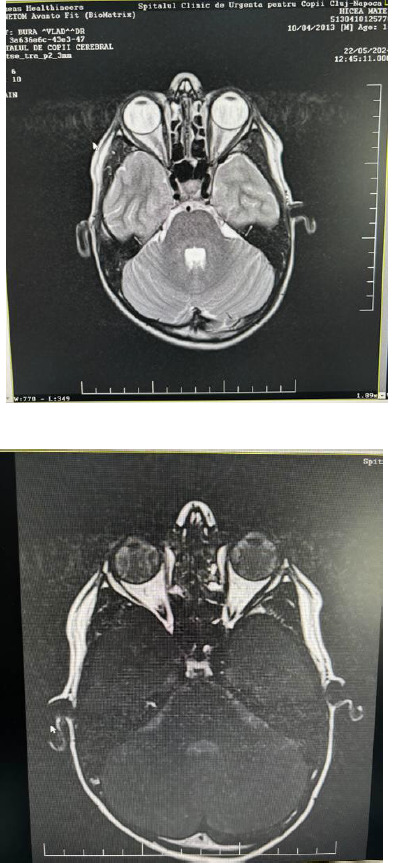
The nuclear magnetic resonance images - normal limits
